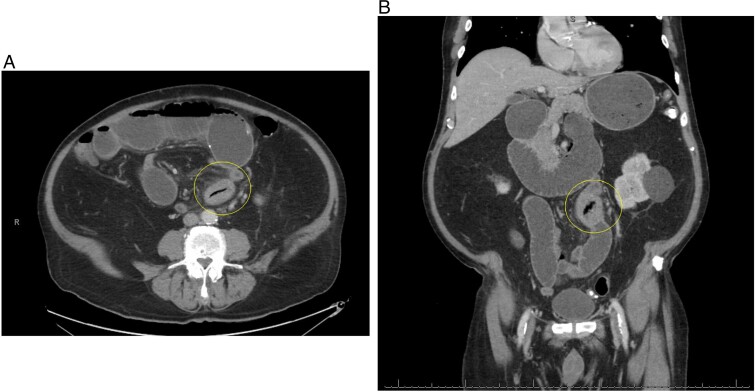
(A) Repeat CT scan (transverse view) on readmission revealing the same primary enterolith with the core pit that mimics intussusception. (B) Repeat CT scan (coronal view) on readmission revealing the same primary enterolith with the core pit that mimics intussusception.
